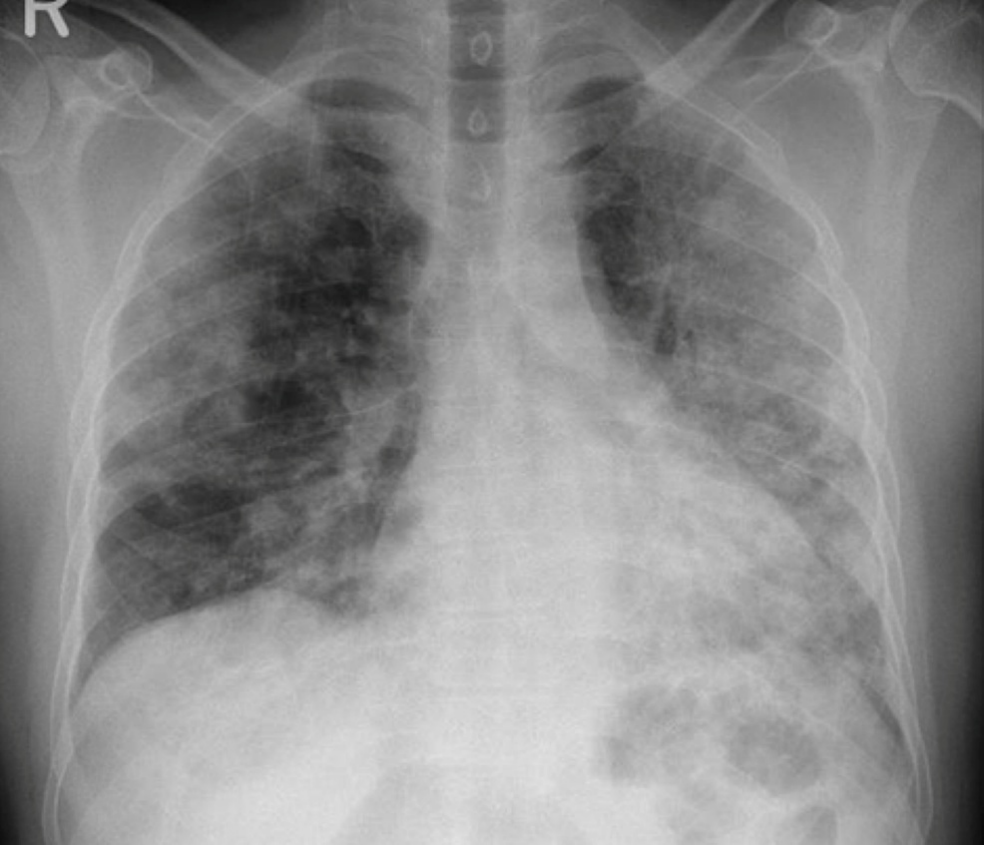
Progression of the bilateral pulmonary consolidation on chest X-ray
